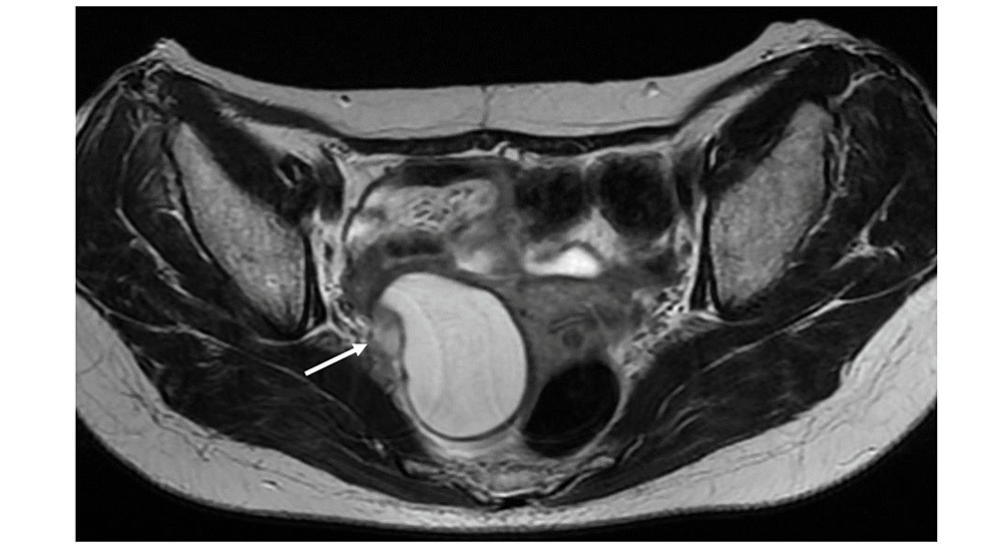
Recurrent tumor An MRI (T2-weighted imaging) performed 42 months after the operation reveals a cystic mass measuring 5.5 cm on the right side of the uterus. The cyst includes an internal protrusion (arrow).
